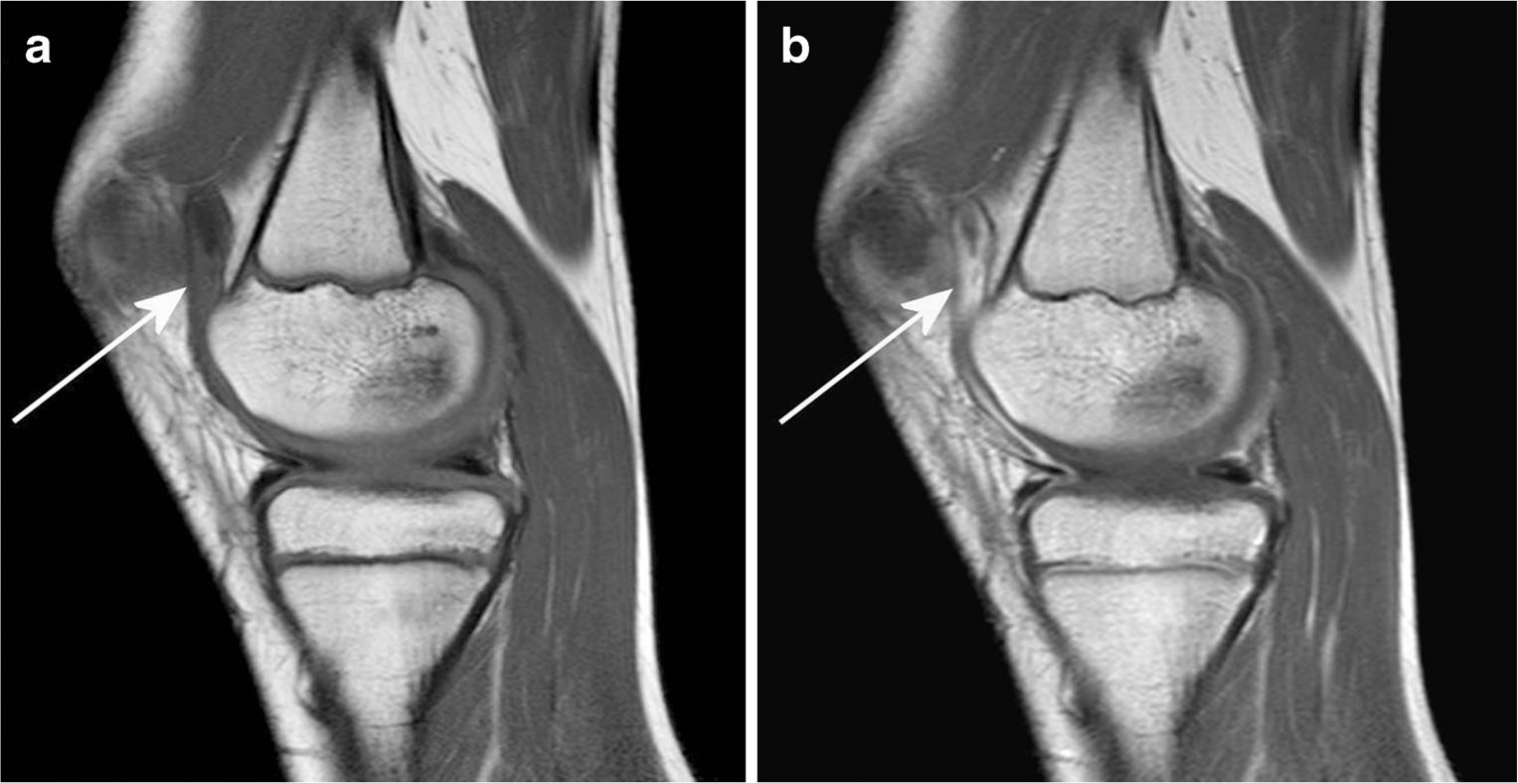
Sagittal T1-weighted MRI sequence (TR/TE 518/10 ms) of the right knee in a 10-year-old girl with clinically inactive juvenile idiopathic arthritis of the oligoarticular subtype. a, b Pre-(a) and post-contrast (b) images show enhancing and thickened synovium at the patellofemoral region (*arrow*)
